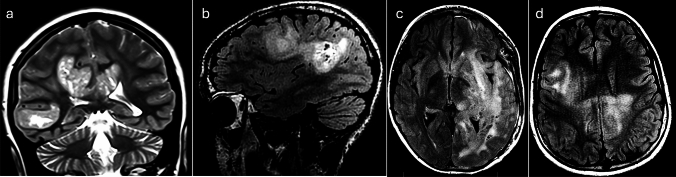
Representative images of four patients (a–d) with gliomatosis cerebri. a Coronal T2 image of patient #5 showed multilobar tumor involvement of the right temporal lobe, right occipital lobe, corpus callosum, and left cingulate. b Sagittal FLAIR image of patient #14 showed multilobar tumor involvement of the left frontal, parietal, and temporal lobes. c Transverse FLAIR image of patient #35 showed multilobar tumor involvement of the left basal ganglia, left thalamus, left side of the splenium, left frontal, left occipital, and left temporal lobes. d Transverse FLAIR image of patient #38 showed multilobar tumor involvement of the bilateral frontal, parietal lobes, corpus callosum, and the left splenium (not shown)
